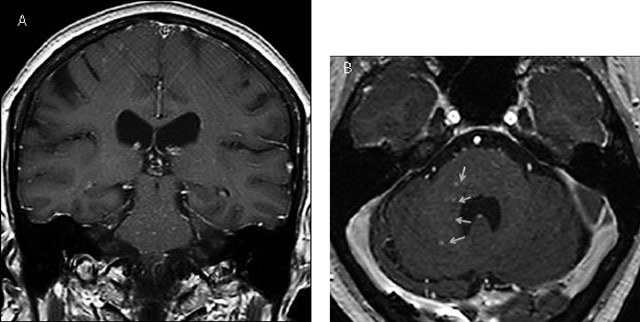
Coronal (A) and axial (B) contrast-enhanced T1 MRI images after 1.5 years showing new punctate and curvilinear lesions predomanintly in the pons and the right middle cerebellar peduncle (yellow arrows).
